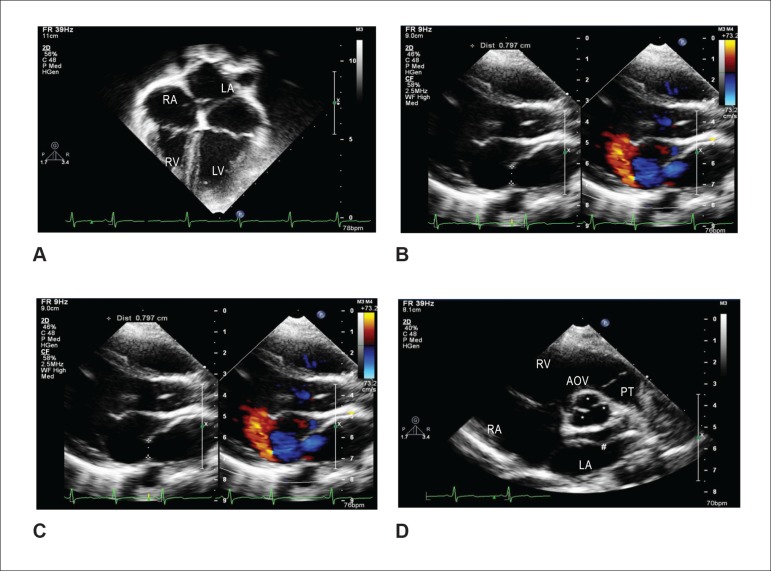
Echocardiograms demonstrate cor triatriatum in the 4-chamber apical plane
